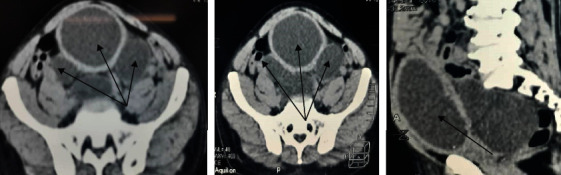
Computed tomographic (CT) scan of the abdomen and pelvis showing multiple bladder diverticula (black arrow).
